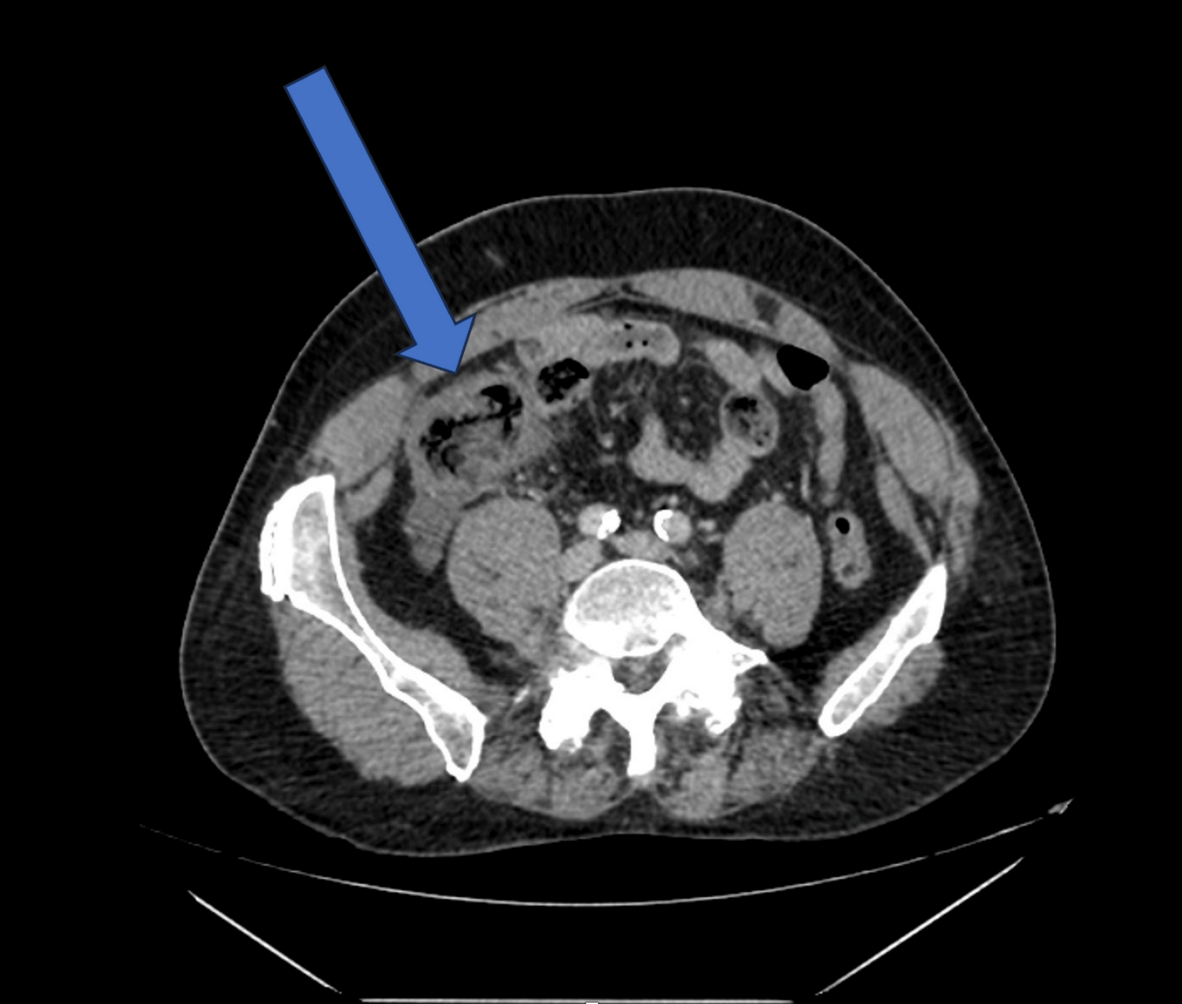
CECT abdomen and pelvis. The arrow highlights the cecal wall, which appears thickened and hypoenhancing with surrounding fat stranding, consistent with ischaemia CECT: Contrast Enhanced Computed Tomography
